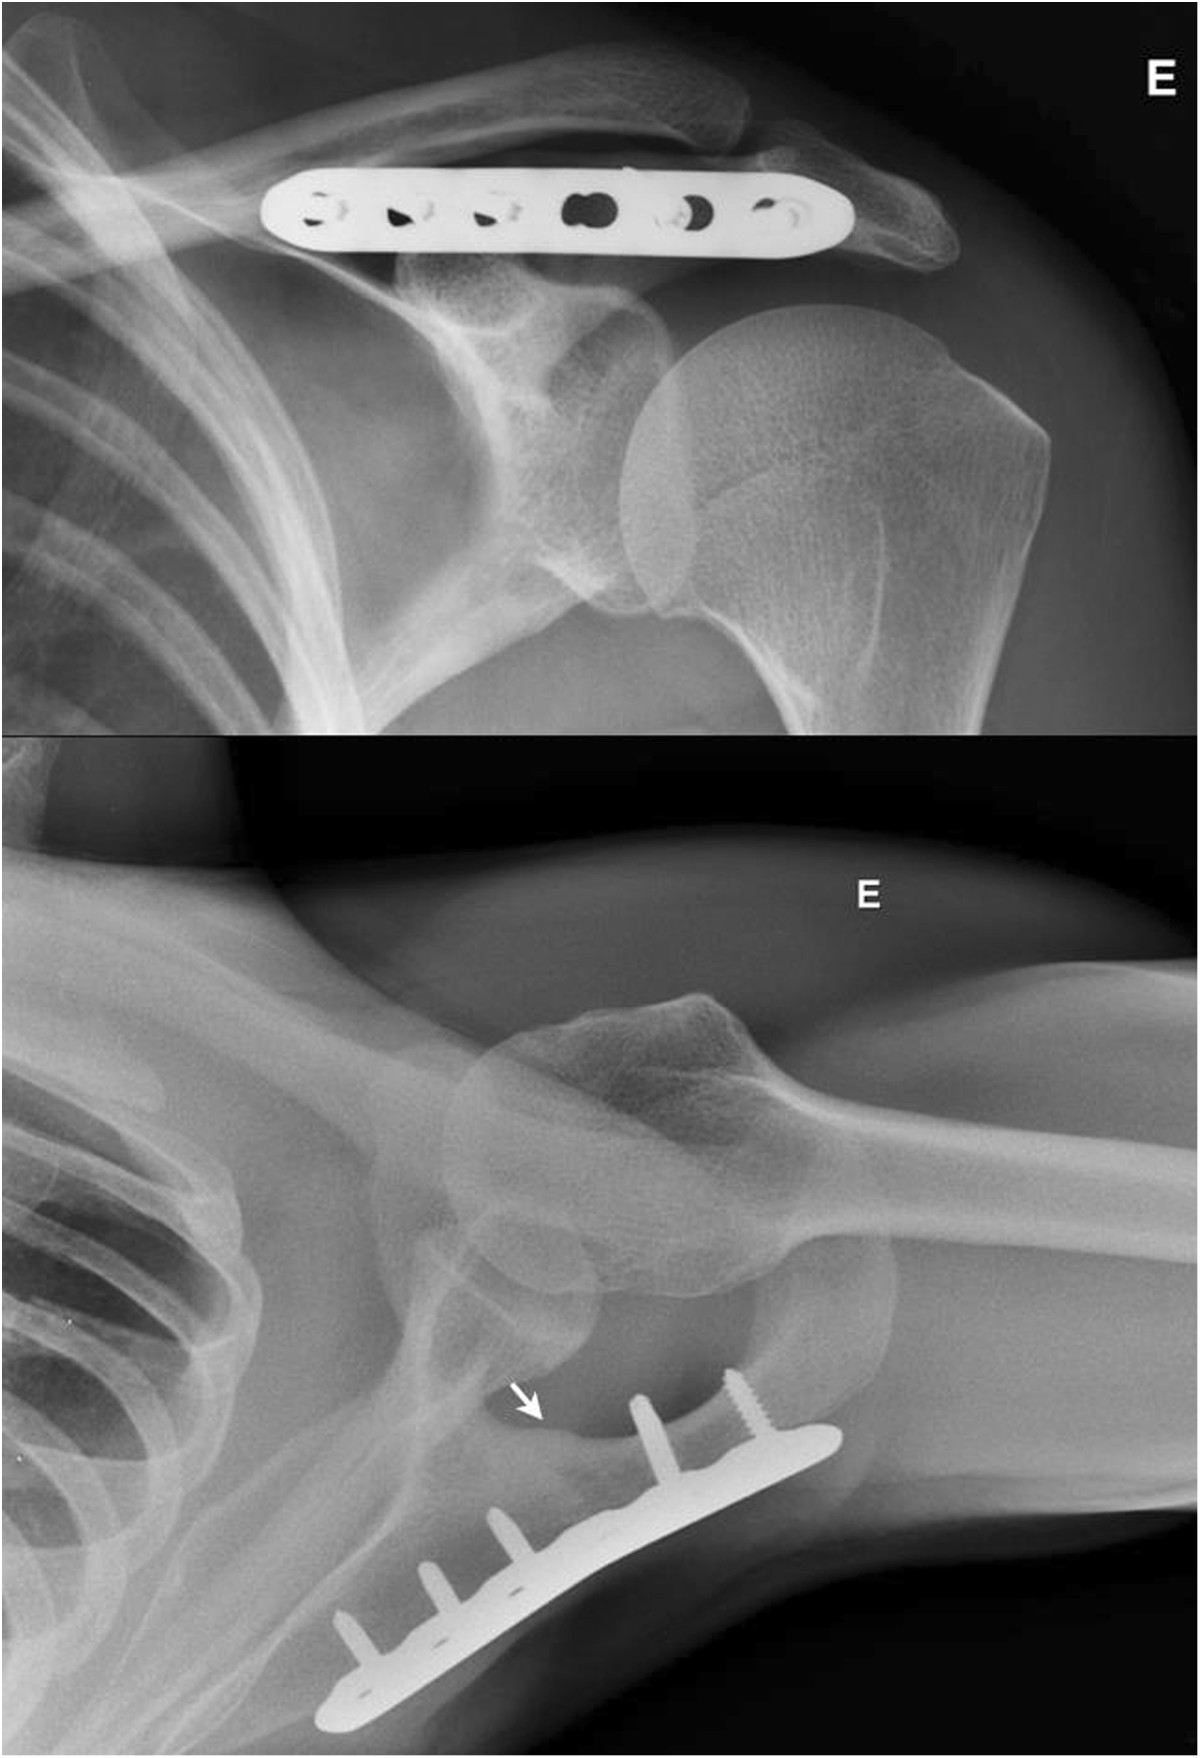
Postoperative radiographs. Postoperative anteroposterior and axillary radiographs demonstrating fracture consolidation (arrow).
